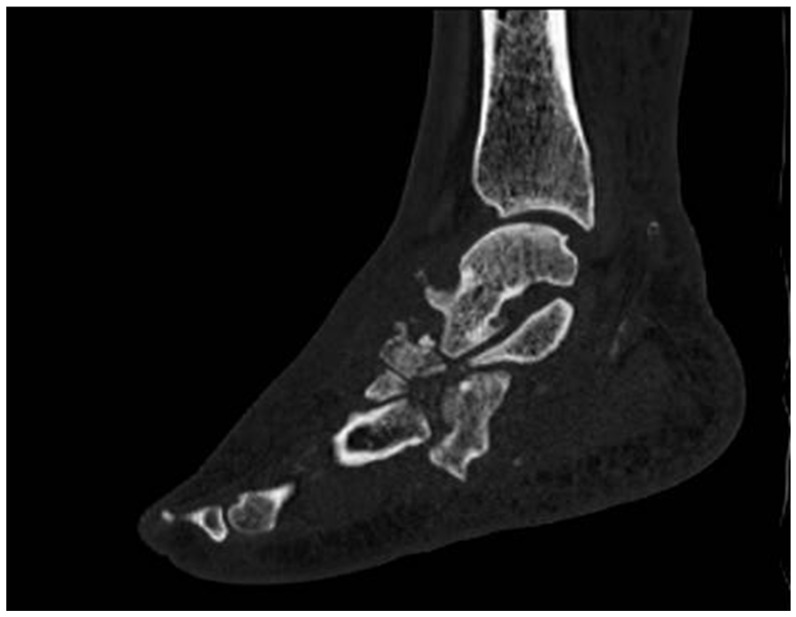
CT scan: Dislocations, bone fragmentation, and structural deformities.
